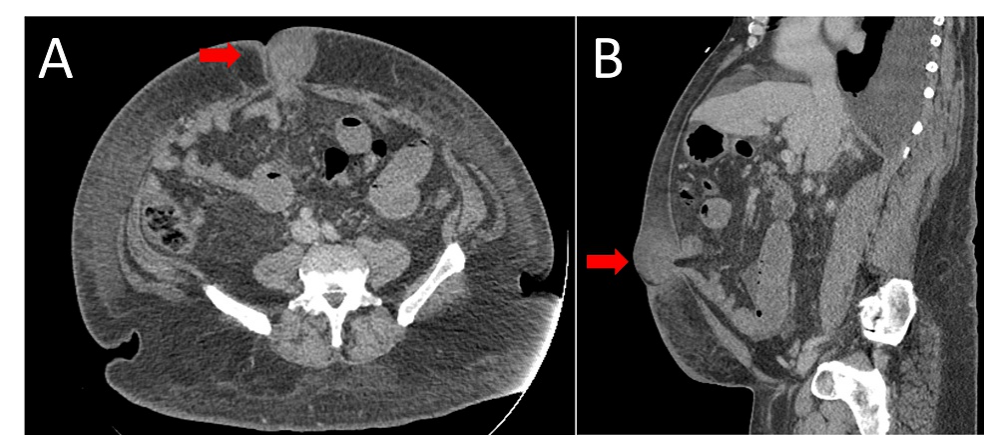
CT abdomen without contrast demonstrating umbilical hernia denoted by red arrows. 1A: Axial view; 1B: Sagittal view.
